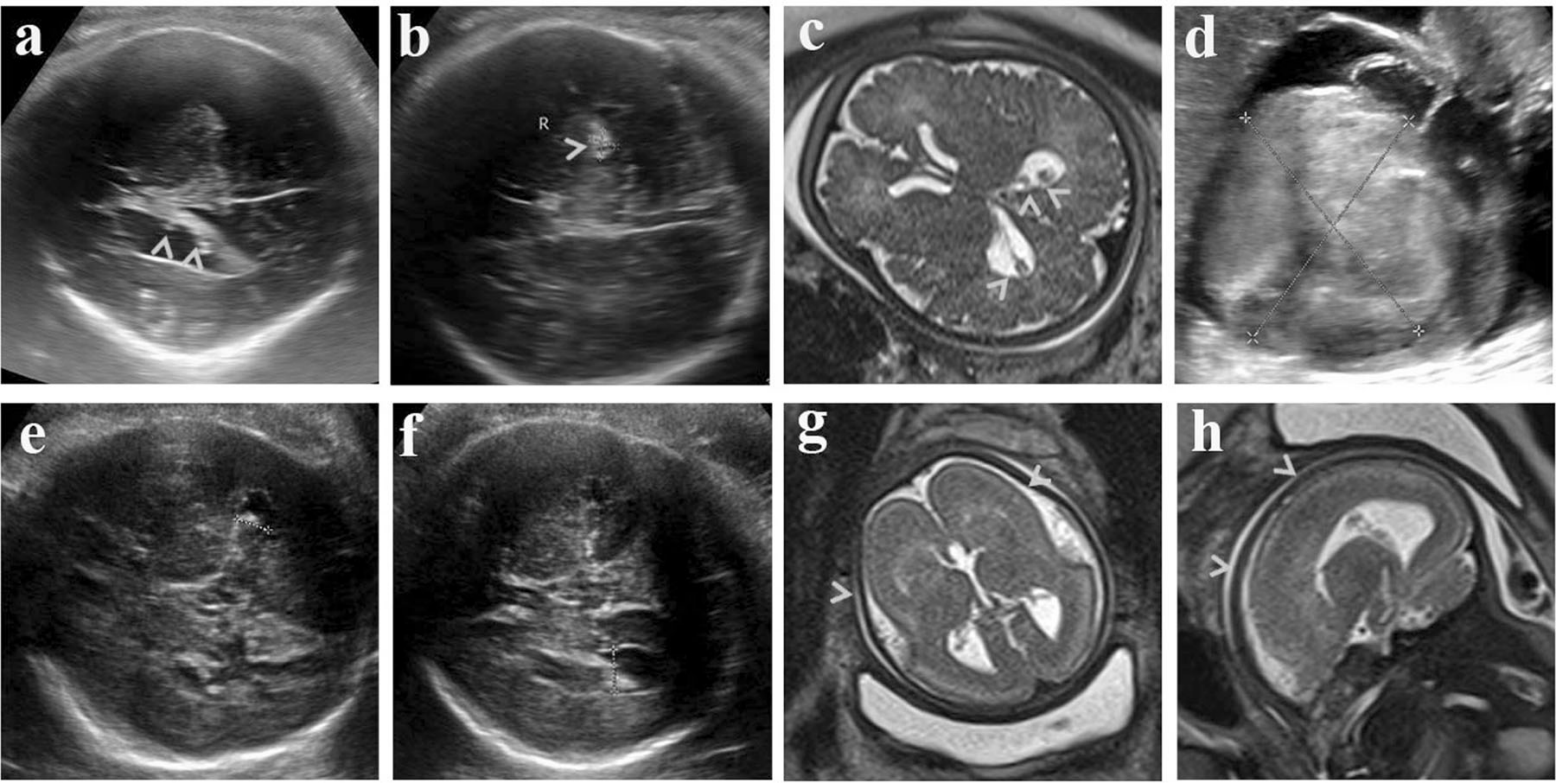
Prenatal imaging of two typical cases, P221 (top row) and P796 (bottom row). Sonographic hyperechoic lesions in a cerebral lateral ventricles and b brain parenchyma indicated by arrows. c Hypointensity lesions revealed by T2-weighted MRI in cerebral lateral ventricles indicated by arrows. d Large space-occupying lesion in left ventricle shown by d sonography. Widths of the cerebral ventricle of P796 were 0.84 cm on left (e) and 1.04 cm on right (f) shown by sonography. g Coronal and h sagittal view of T2 MRI showing abnormal cortical gyration indicated by arrows.
